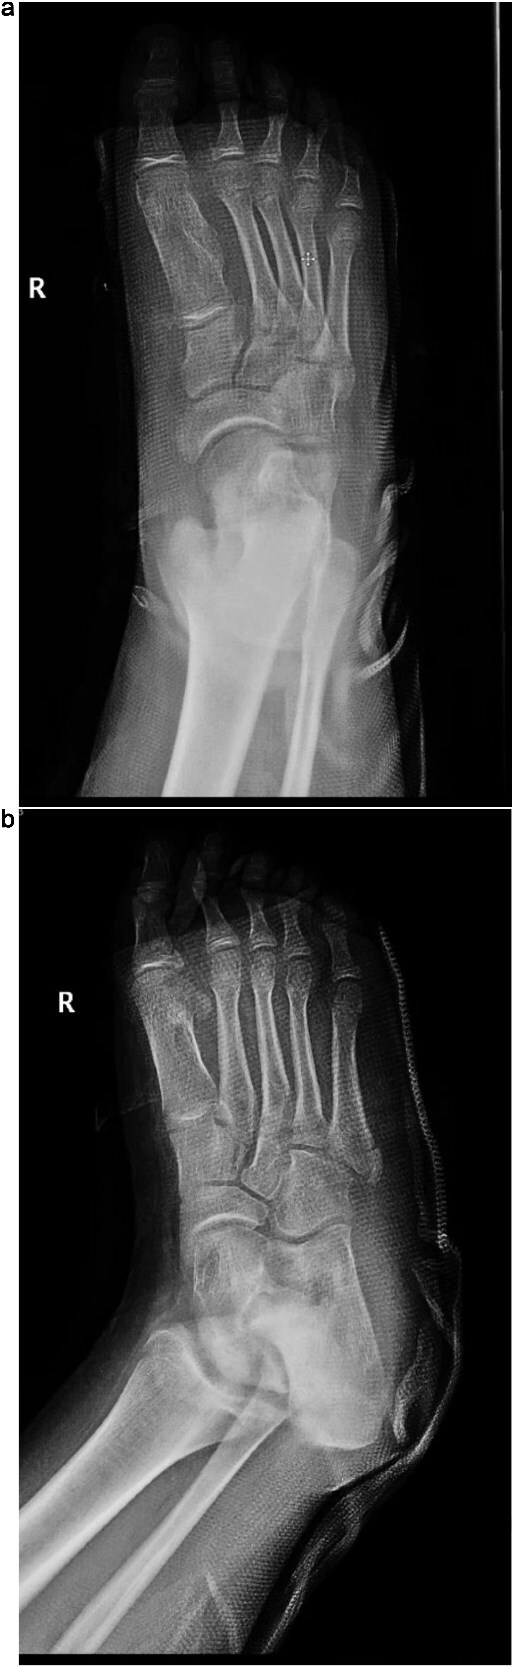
(a) Postoperative anteroposterior radiograph of the right foot demonstrating complete resection of the intermetatarsal lesion and normalization of the intermetatarsal spacing. (b) Oblique postoperative view confirming successful removal of the mass with preserved alignment of the first metatarsal and surrounding structures.
